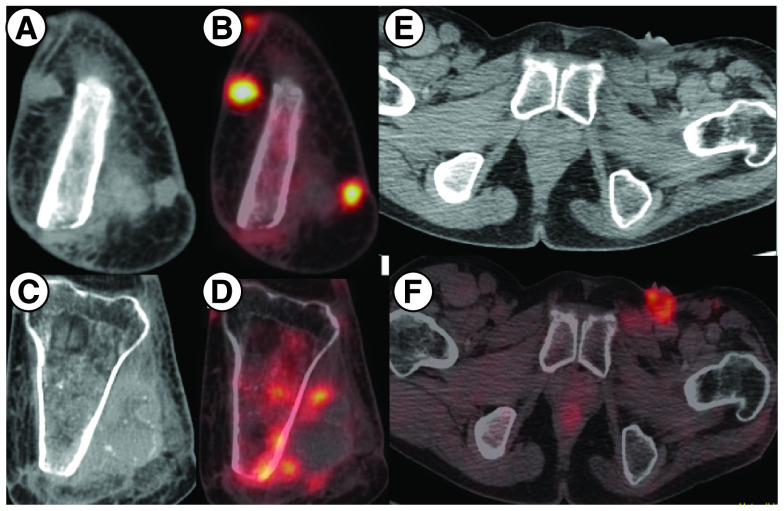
PET scan imaging showing radiographic findings prior to starting tazemetostat. (A & B) MIP image showing focal increased tracer uptake in the left inguinal region and the left knee joint region corresponding to cutaneous nodules at the amputated site of left tibia and fibula on CT showing increased FDG uptake on fused PET-CT. (C & D) Areas of sclerosis in the left tibia with increased FDG uptake. (E–G) Ulcerated left inguinal lymph nodes on CT showing increased FDG uptake on fused PET-CT. CT: Computed tomography; MIP: Maximum intensity projection.
